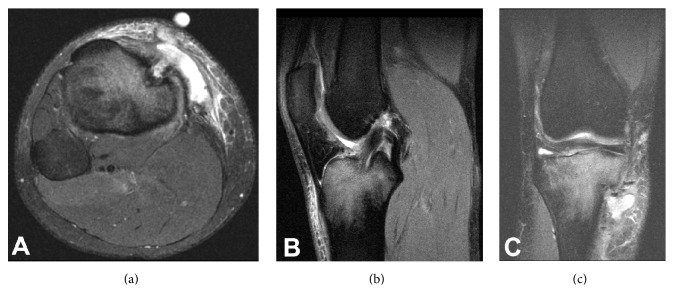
(a) Axial slice of the MRI demonstrating the pretibial fluid collection in continuity with the orifice of the tibial tunnel. (b) T2 sagittal image demonstrates an intact ACL graft with adjacent tibial bone marrow edema. (c) T2 coronal image again demonstrates the pretibial fluid collection in continuity with the tibial tunnel and proximal tibial edema.
